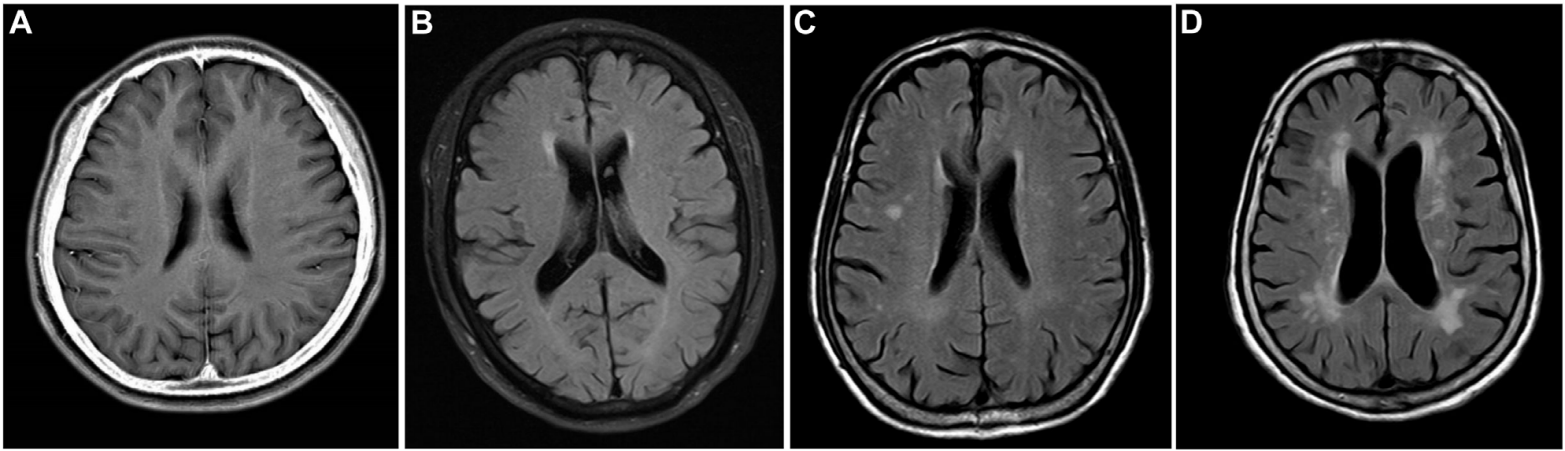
MRI T2 FLAIR image. Grade 0: no white matter lesions (A). Grade 1: focal white matter lesions (B). Grade 2: partial fusion of white matter lesions (C). Grade 3: diffuse involving the entire area (D).
